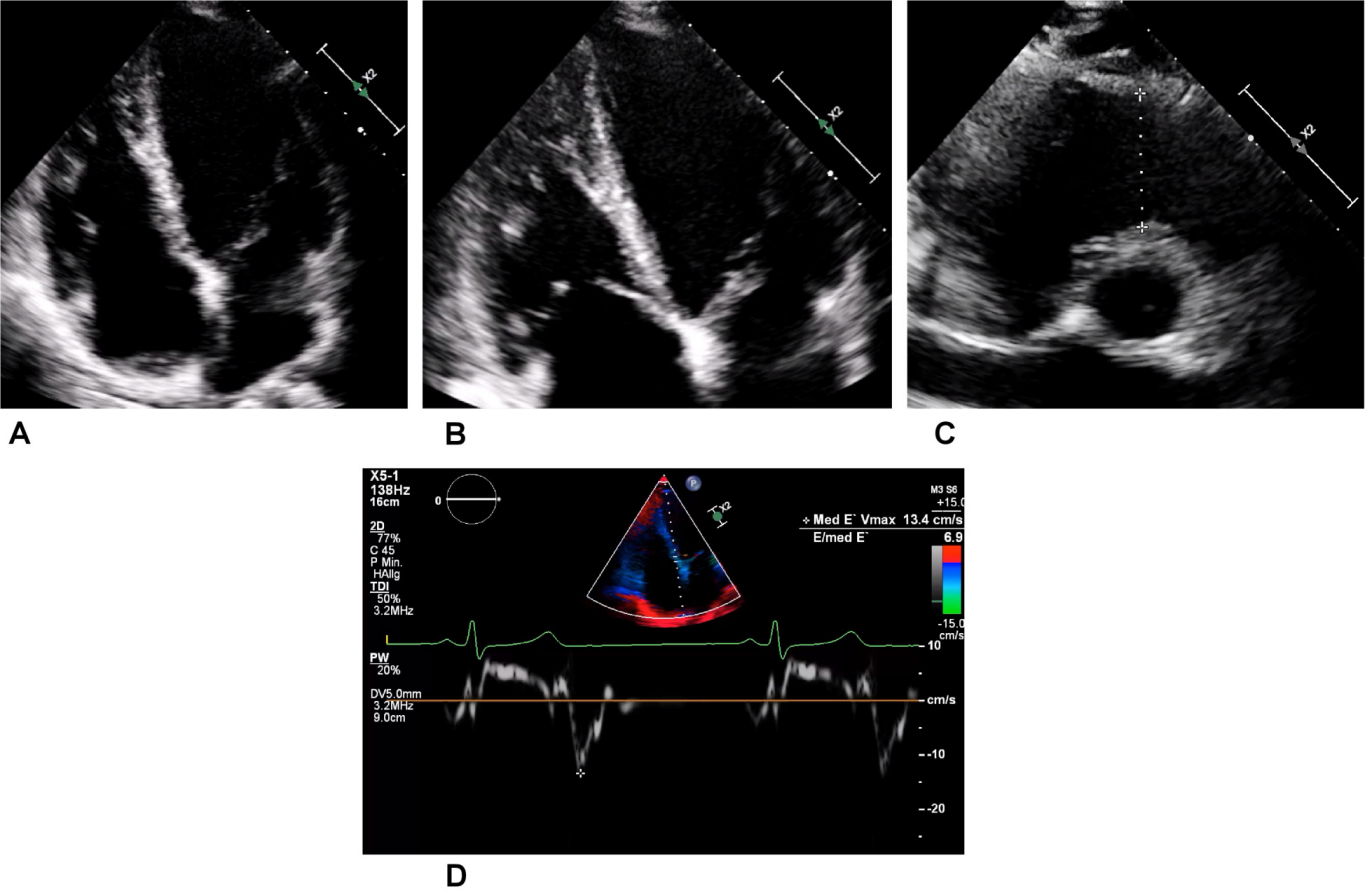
Transthoracic echocardiography of the asymptomatic athlete. (A) Regular four-chamber view. (B) Right dominant four-chamber view showing a trabeculated free wall in the right ventricle. (C) Slight dilatation of the right ventricular outflow tract (a distance of 34 mm is represented by the dotted line) in this normally sized young woman. (D) Medial tissue Doppler imaging revealing regular velocity.
